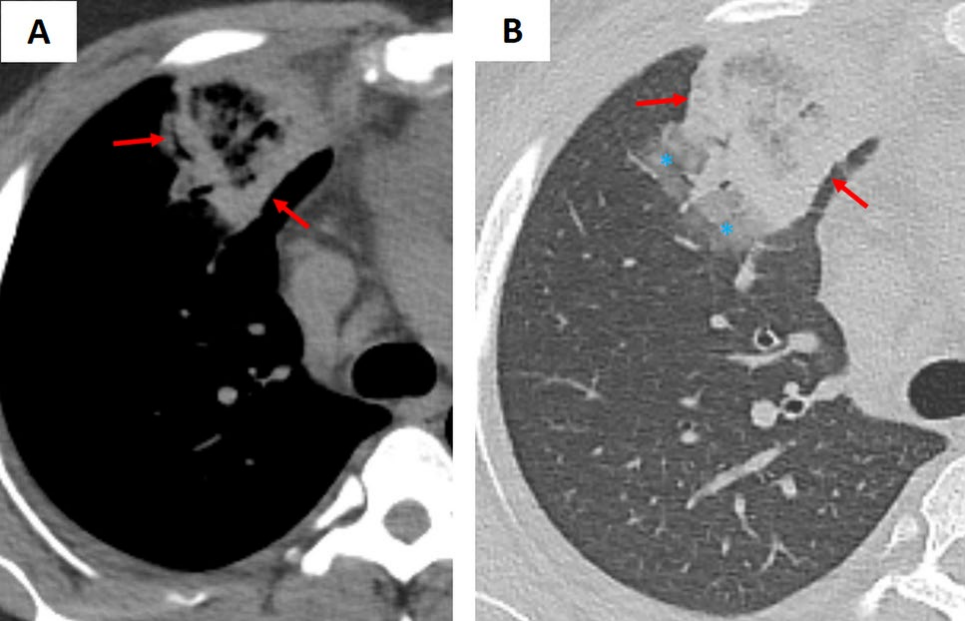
Atoll sign (arrows) in a patient with colon carcinoma and presented with cough and fever. The patient diagnosed with septic pulmonary embolism due to *Bacteroides fragilis* septicemia. Axial (A) mediastinal and (B) lung window CT images demonstrate a solitary, right upper lobe anterior wedge-shaped opacity with the atoll sign. Note the surrounding ground-glass opacity (*), indicative of associated inflammation, hemorrhage, or infarction
